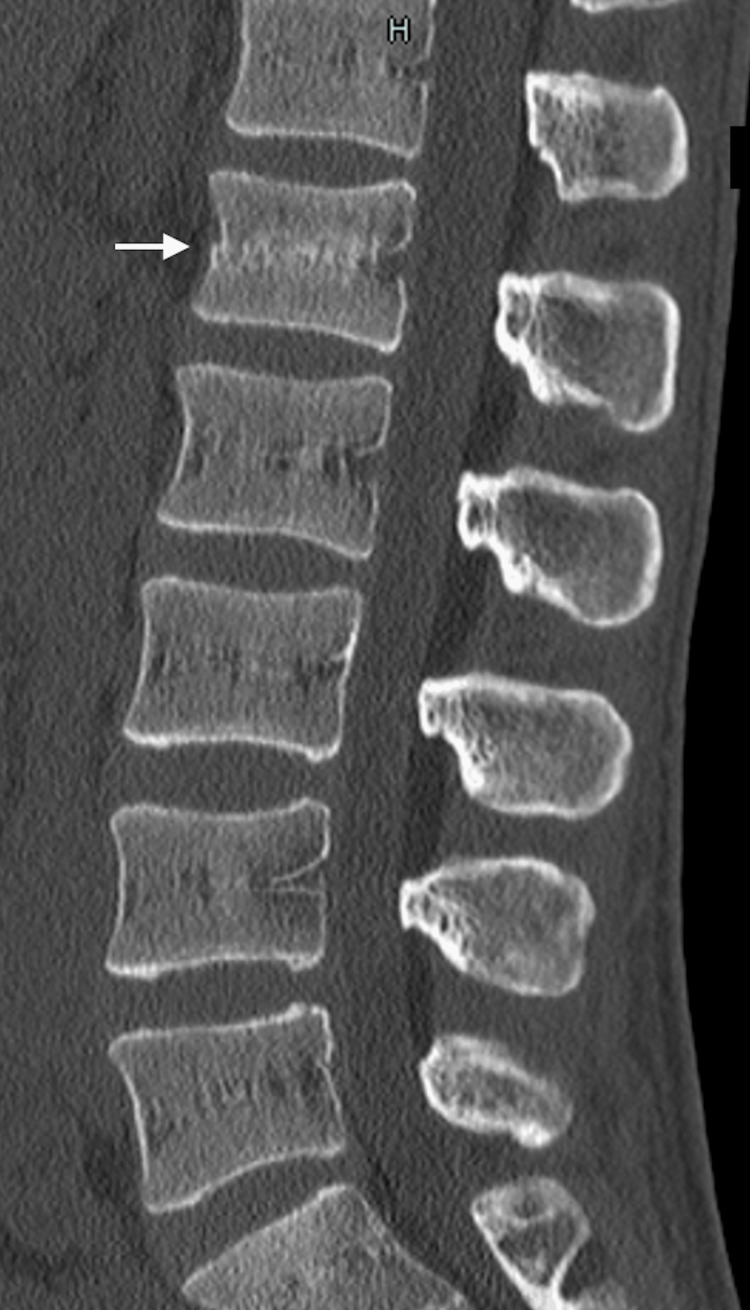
Findings at 10 weeks after injury CT sagittal view of the spine showing vertebral bone union (white arrows). CT: computed tomography
